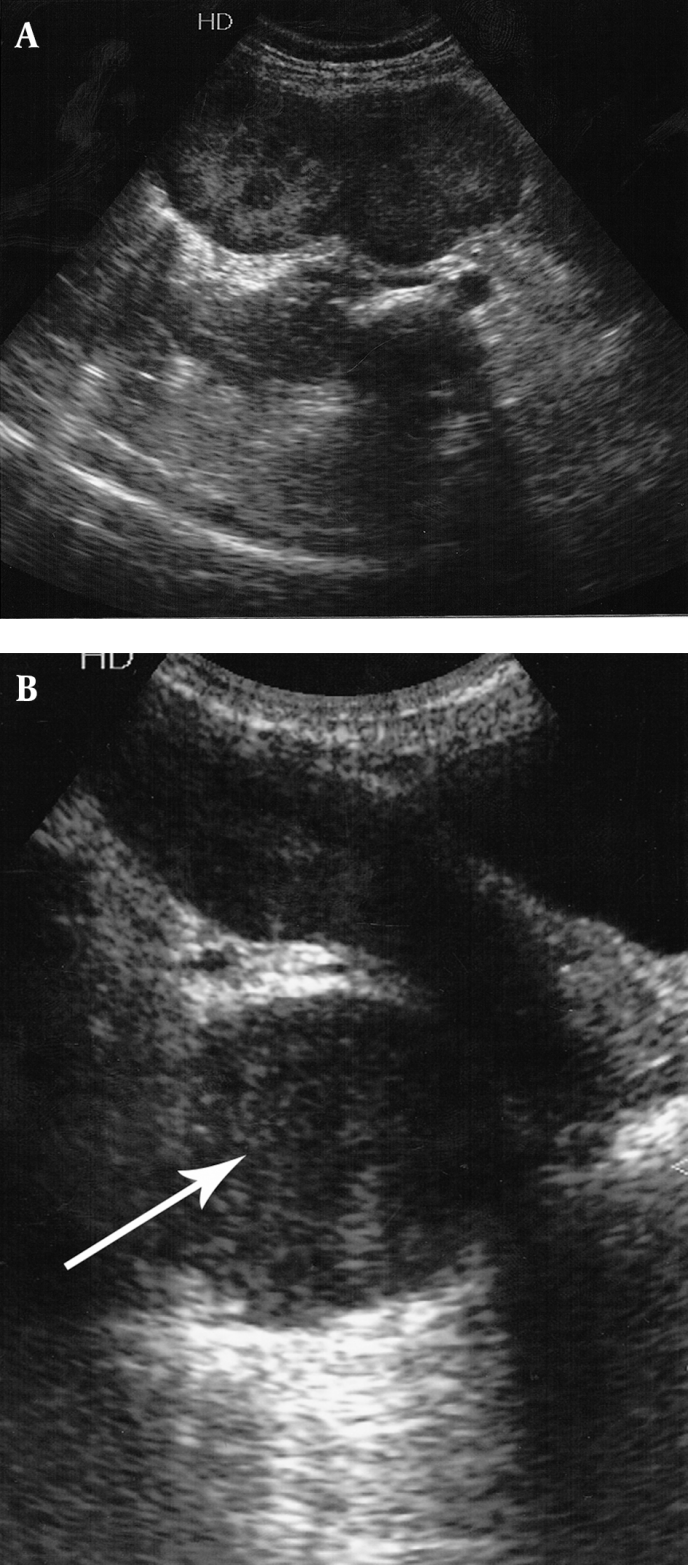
Ultrasonography of the abdomen and pelvis demonstrating well-defined hypoechoic lesions in the A, right paraspinal and B, the presacral regions.
